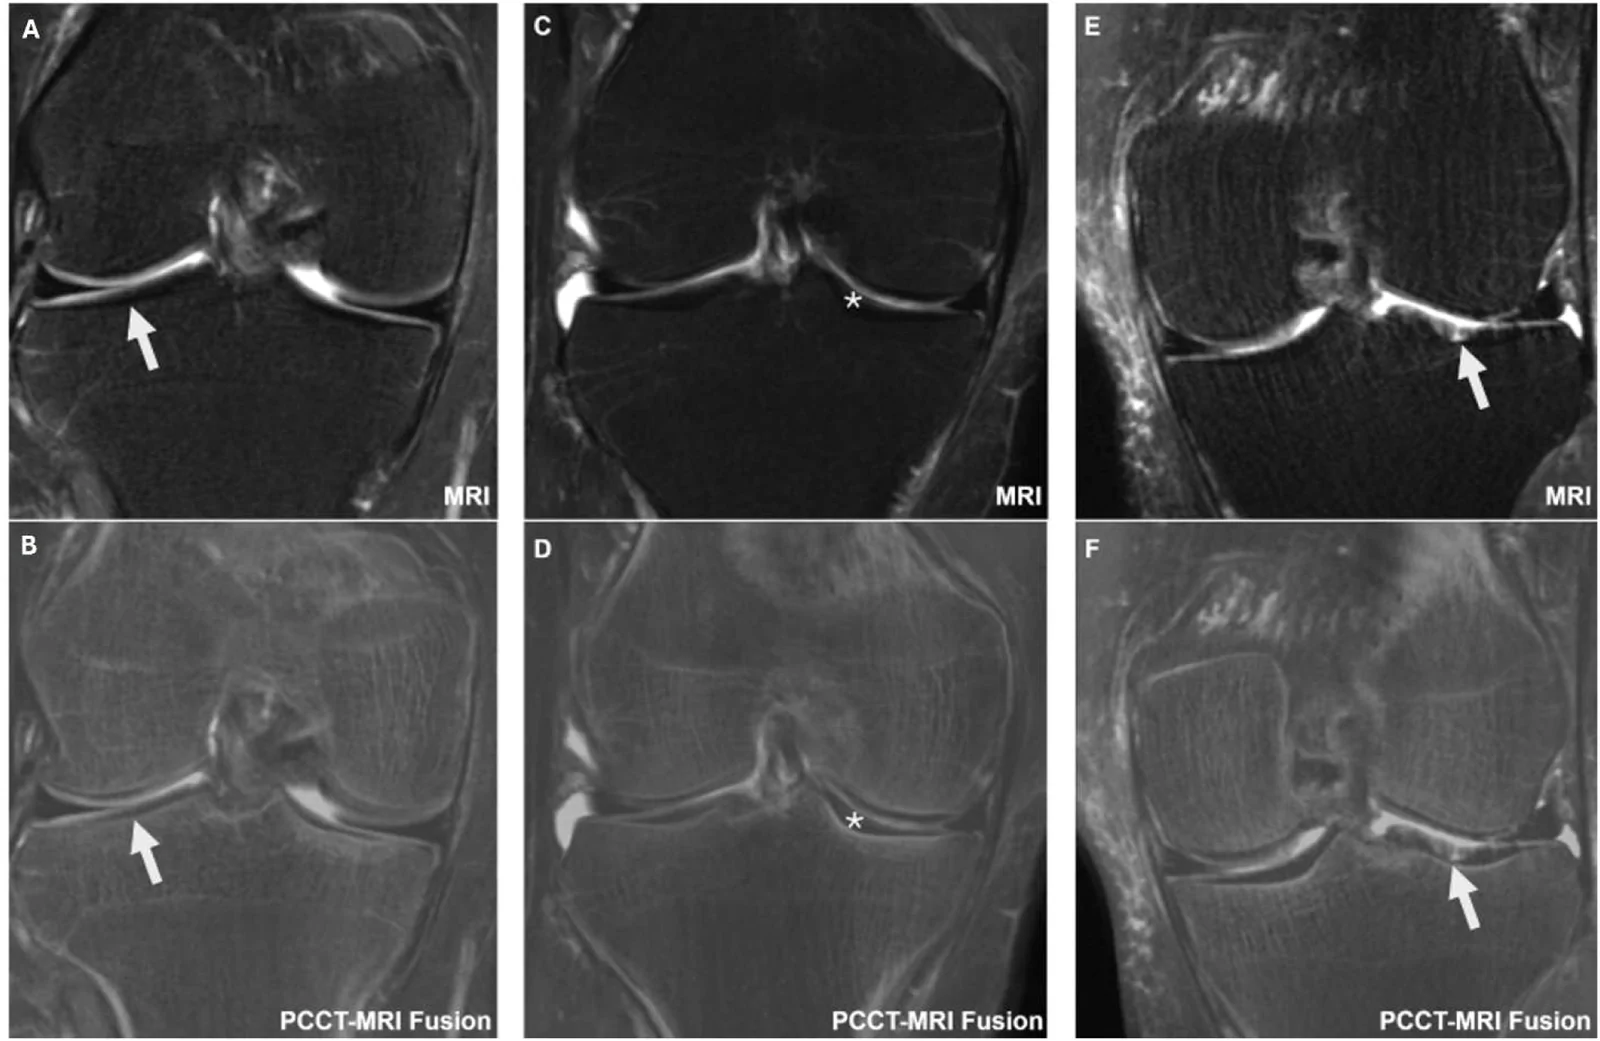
Representative examples comparing MRI alone (upper row) and PCCT-MRI fusion (lower row) images. (A) Coronal T2-weighted MRI with fat-suppression with normal tibial and femoral cartilage thickness and surface. (B) The PCCT-MRI fusion image shows a much better delineation of the subchondral bone plate (arrow) compared to the MRI alone. (C) Coronal T2-weighted MRI with fat-suppression showing thinning of the tibial and femoral cartilage. The precise thickness of the femoral compartment cartilage is difficult to assess given the low T2 signal of the deep cartilage layer. (D) The PCCT-MRI fusion image shows a much clearer delineation of the subchondral bone plate (star) allowing a more accurate assessment of the degree of cartilage loss. (E) Coronal T2-weighted MR image with fat suppression showing focal high-grade cartilage loss of the medial tibial plateau (arrow). (F) The combined image shows a focal irregularity of the underlying subchondral bone plate with a remaining layer of deep cartilage.
